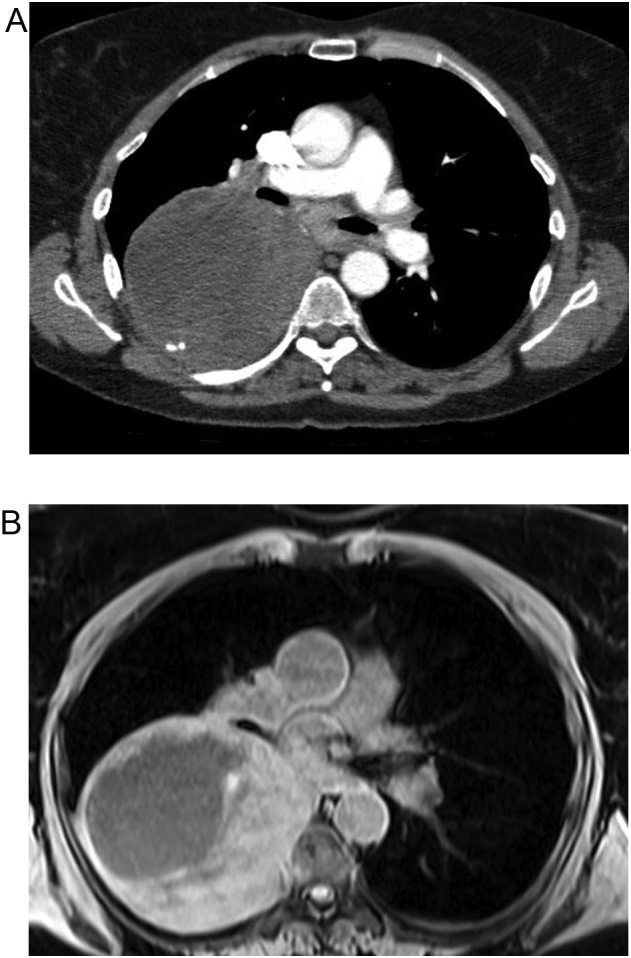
A: Computed tomography of the chest demonstrating a 13 cm pleural-based mass with focal areas of necrosis and arterial enhancement. B: Magnetic resonance imaging of the chest demonstrating focal extension of the mass through the parietal pleura into the paravertebral space and into the T7 vertebral body without encroachment into the spinal canal or evidence of spinal cord compression.
